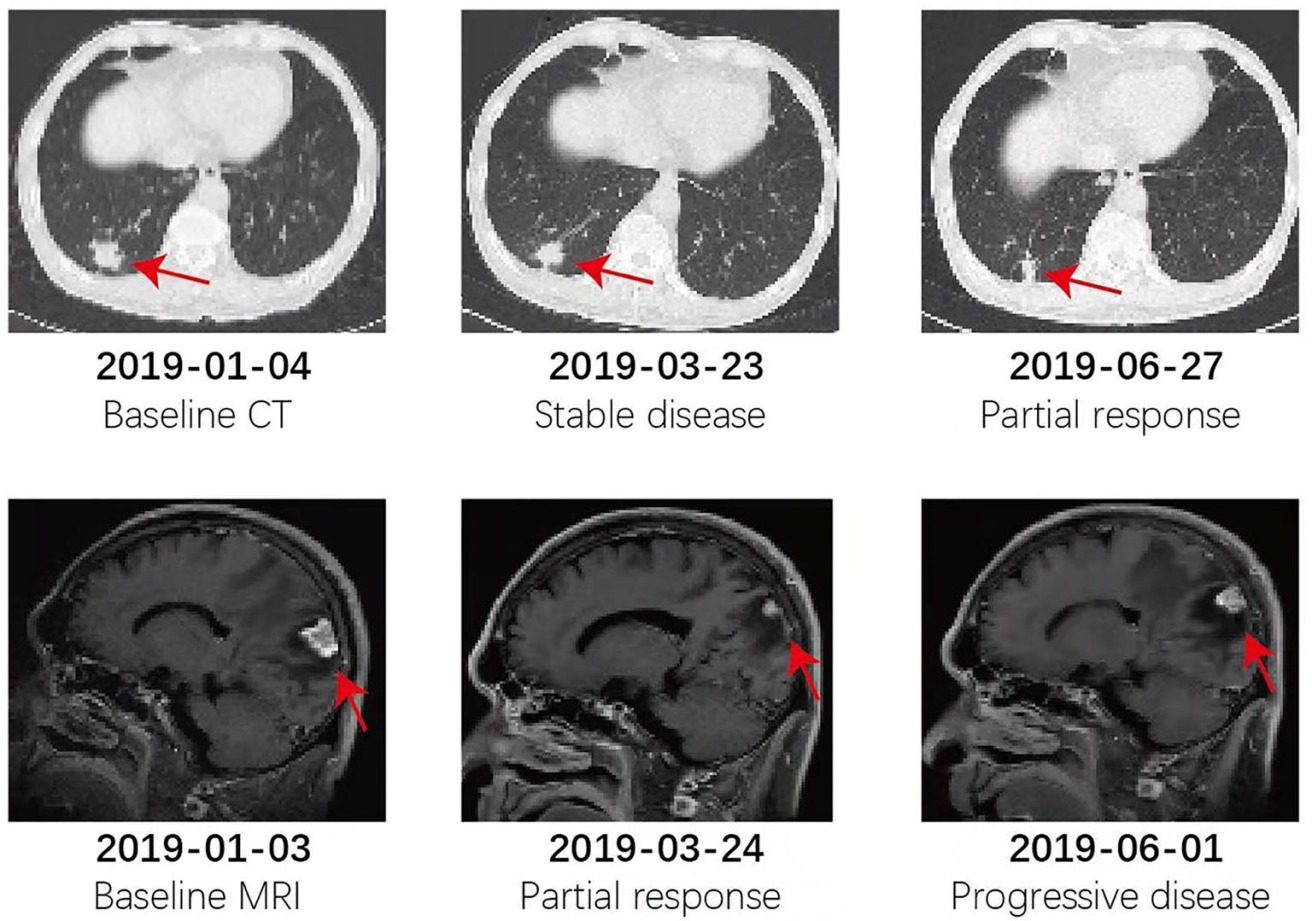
Patient dynamic changes in the chest CT and brain MRI during treatments.
